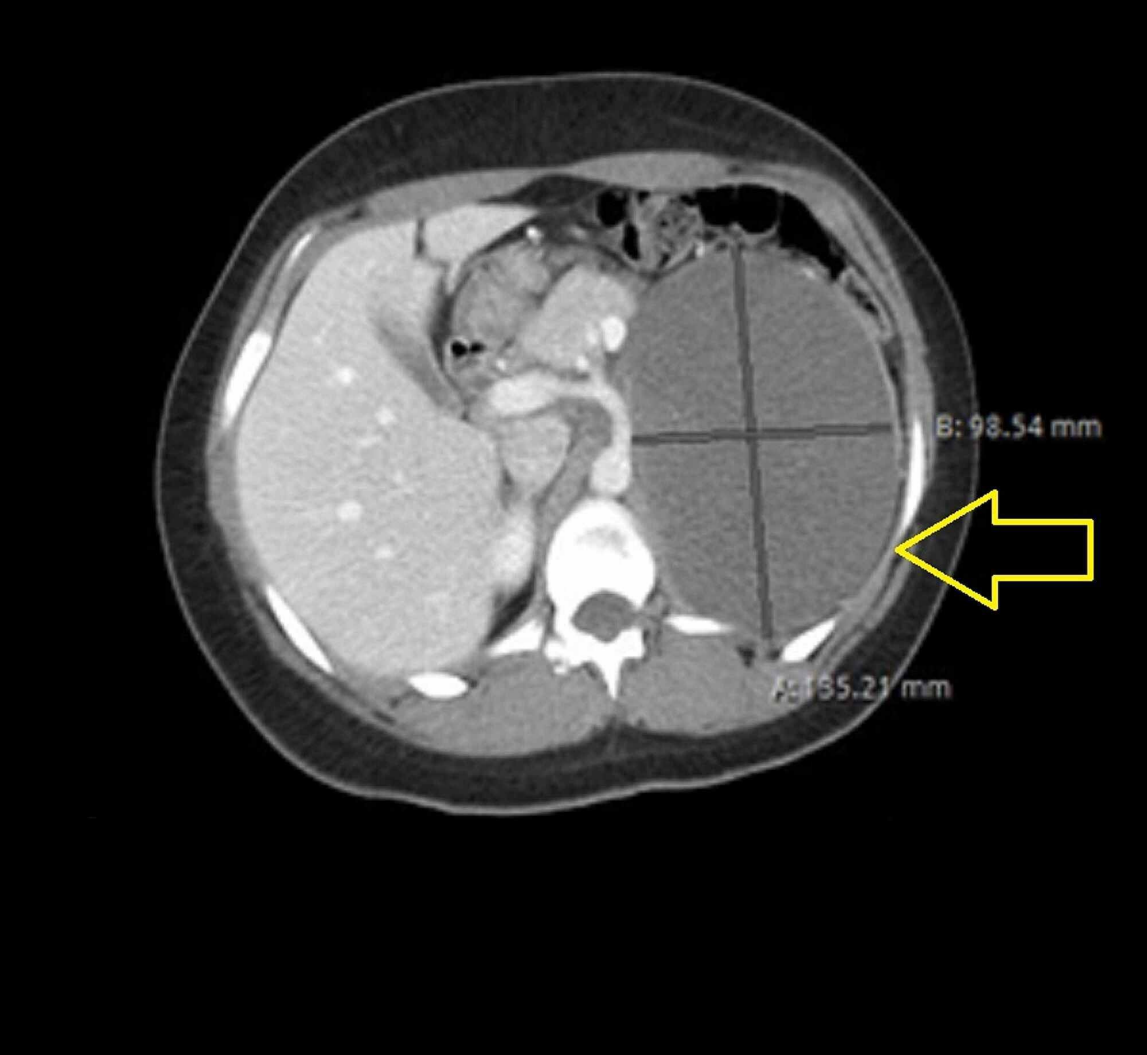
CT abdomen/pelvis axial view. The yellow arrow demonstrates a large mass causing displacement of adjacent structures.
